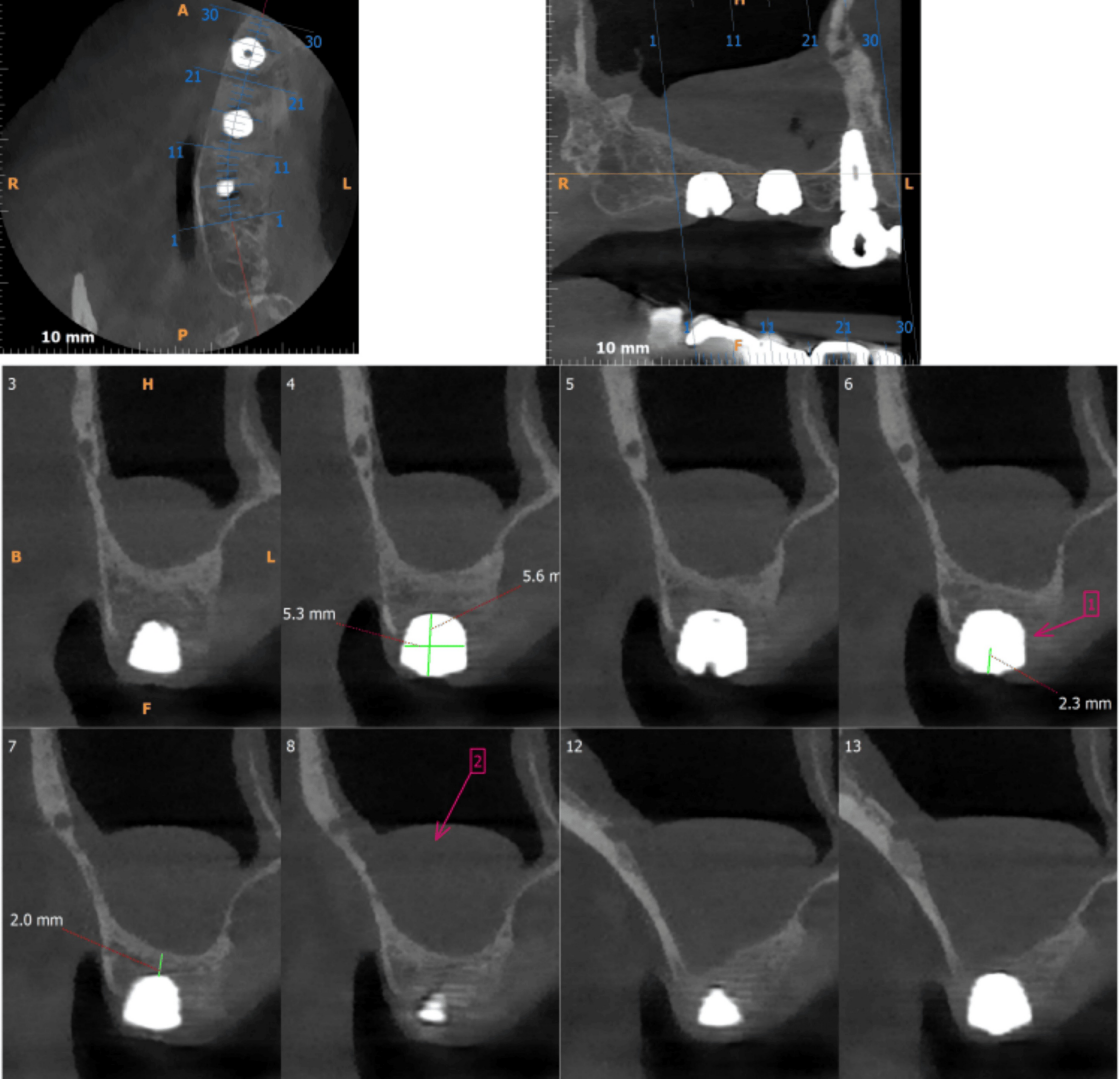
Six-month postoperative cone beam computed tomography showing the osseointegrated implant in region 16 with sound bone surrounding the implant.
